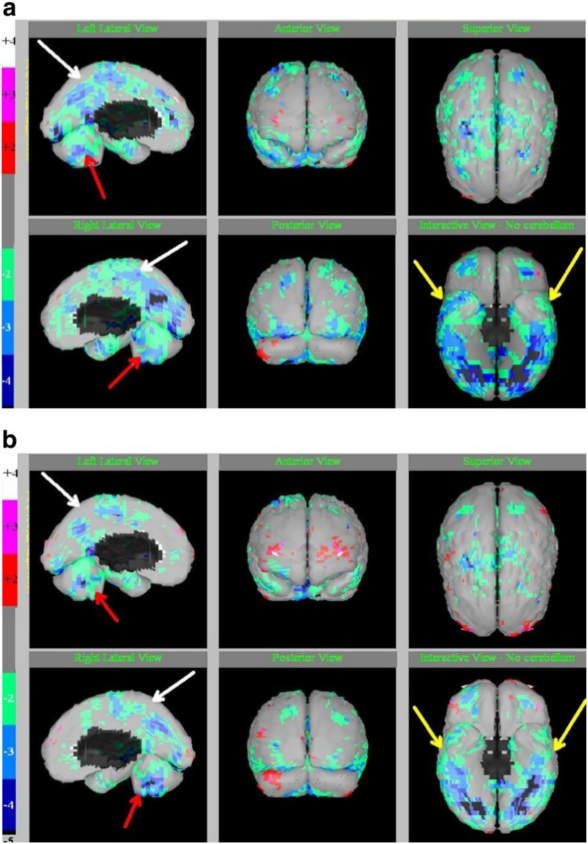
SPECT scan images in a 12 year old boy with autism (a) before and (b) after 80 sessions of HBOT at 1.3 atm. Legend: minus 2 (green) to minus 4 (blue) standard deviations indicate the magnitude of regional hypofunctioning (hypoperfusion). White arrows indicate improvement in deeper cortical hypoperfusion patterns. Red arrows on sagittal slices show the midline cerebellum hypoperfusion and improvements after HBOT. Yellow arrows on the “underside” view show the temporal lobe hypoperfusion with improvements after HBOT. Pictures courtesy of J. Michael Uszler, MD. Credit: Permission for use of figure from Hyperbaric Oxygen for Neurological Disorders granted by Best Publishing Company, Palm Beach Gardens, FL.
